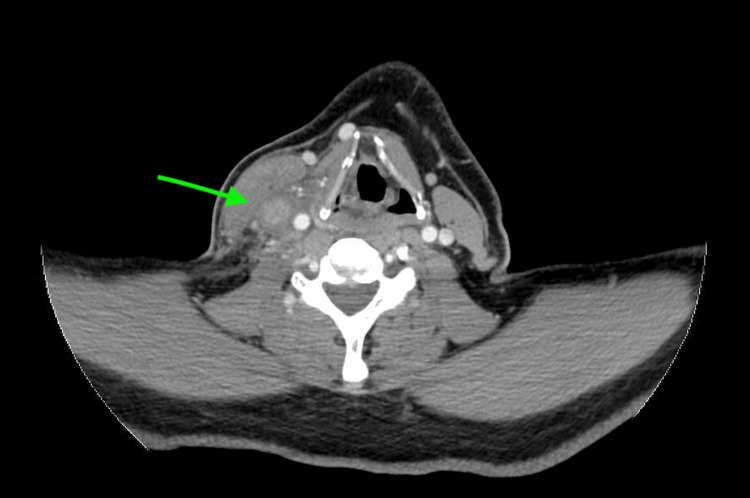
Axial section obtained from CT of the neck with intravenous contrast demonstrates a sudden loss of contrast opacification in the right internal jugular vein, accompanied by surrounding stranding and edema, suggesting thrombophlebitis with no discernible fluid collection amenable to drainage (green arrow).
